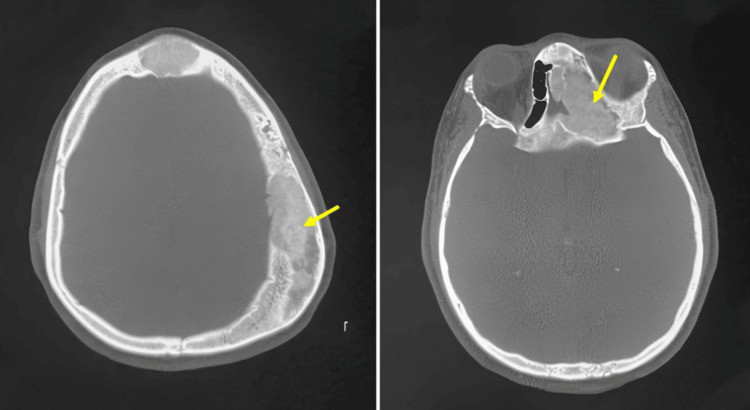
Axial cranial CT scan on bone windows showing a diploe hypertrophy with thinning of the internal and external tables, with a ground-glass appearance involving the frontoparietal bone (arrow) and the frontal and ethmoid sinuses (arrow).
